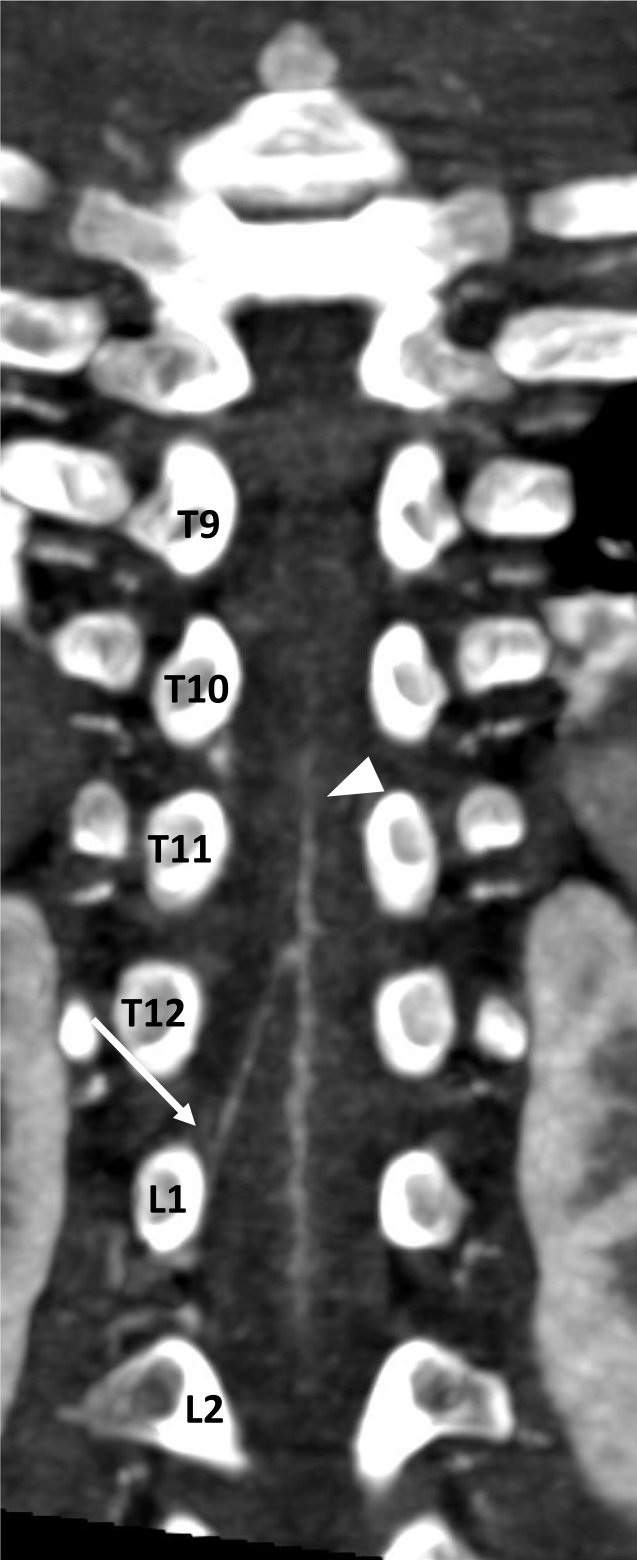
24-month-old male with a body surface area of 0.52 m^2^. Coronal MIP CTA of the chest in soft tissue windows. kV=70, mAs=282. The artery of Adamkiewicz can be seen arising from L1 on the right (*arrow*) feeding the anterior spinal artery (*arrowhead*)
